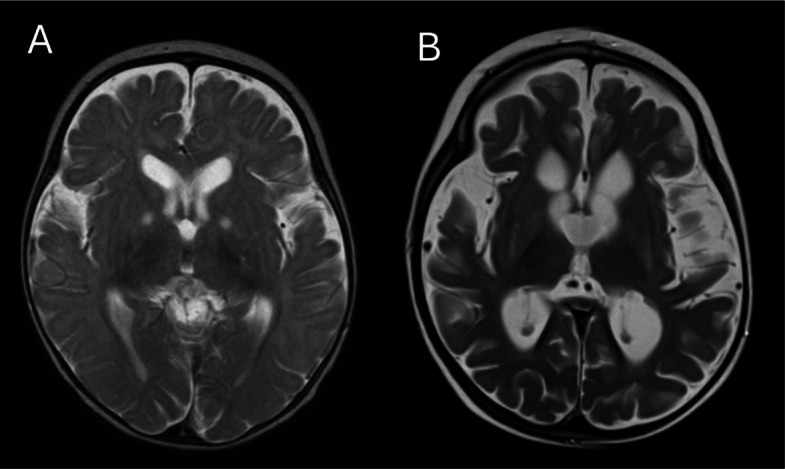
Representative brain MRI at 7 and 20 months of age. Serial MRI-brain imaging at 7 (A) and 20 (B) months of age, showing progressive changes with abnormal myelination, T2-hyperintense pallidal lesions and progressive atrophy
